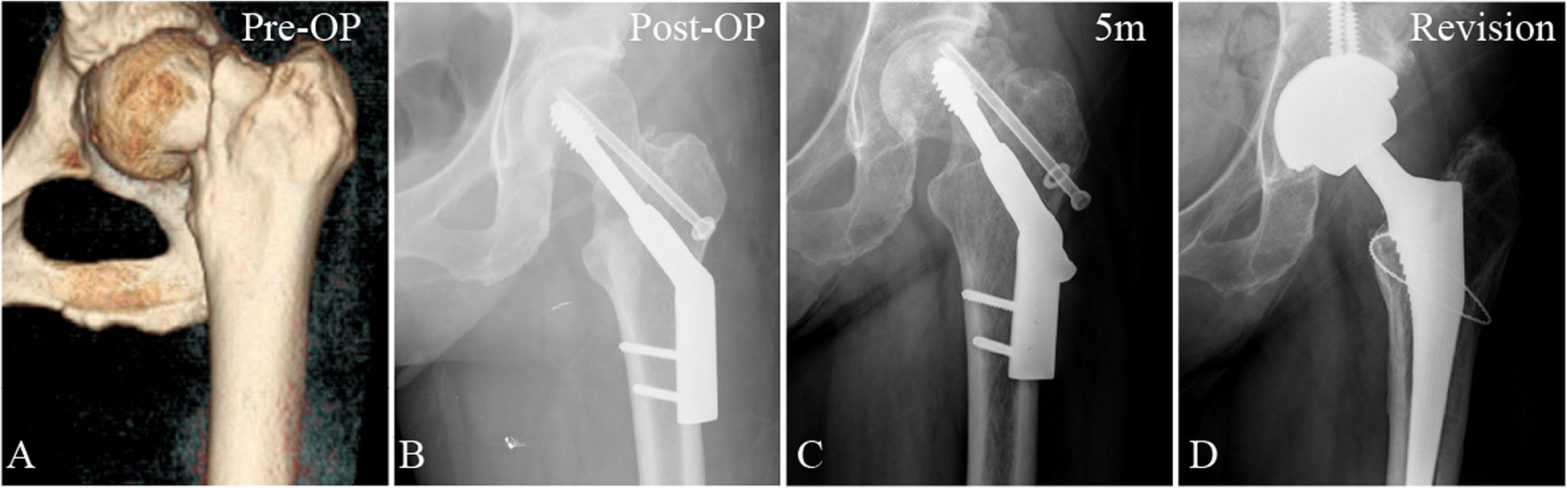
3-D reconstructed model of hip and AP radiographs of fracture, treatment fixation and revision implants in a 59-year-old woman with a vertical femoral neck fracture. a Reconstructed model (AP view) from preoperative CT images showing the location of the fracture. b AP radiograph showing initial treatment with a dynamic hip screw with an anti-rotational screw. c AP radiograph of the same patient taken 5 months after initial surgery, revealing severe screw withdrawal, femoral neck shortening, varus deformation, and delayed union. d AP radiograph of the same patient after revision operation with arthroplasty
